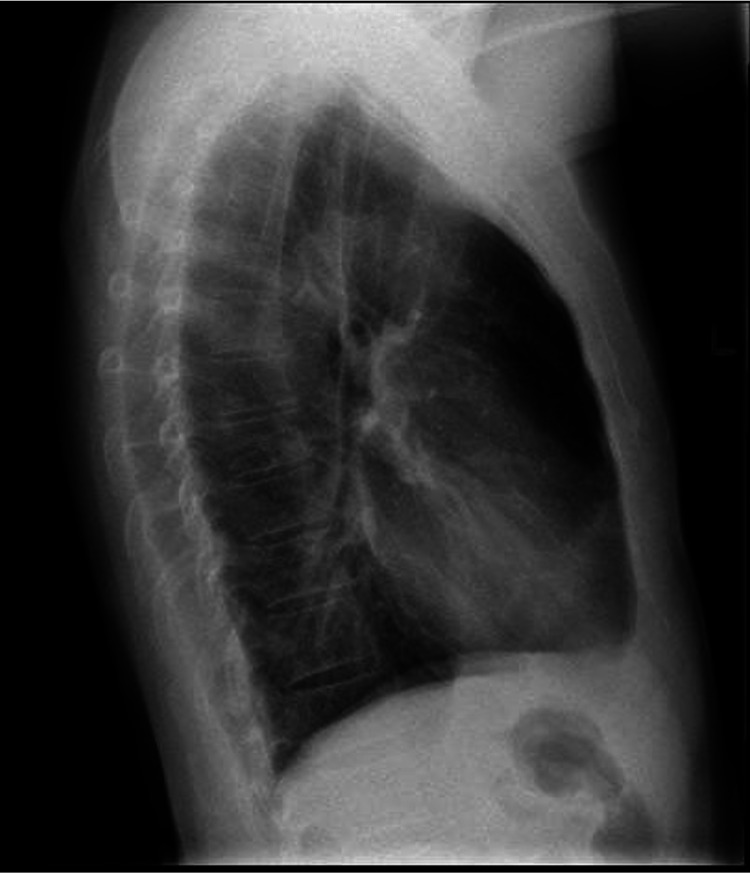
Lateral chest X-ray showing resolution of the left retrocardiac density.
